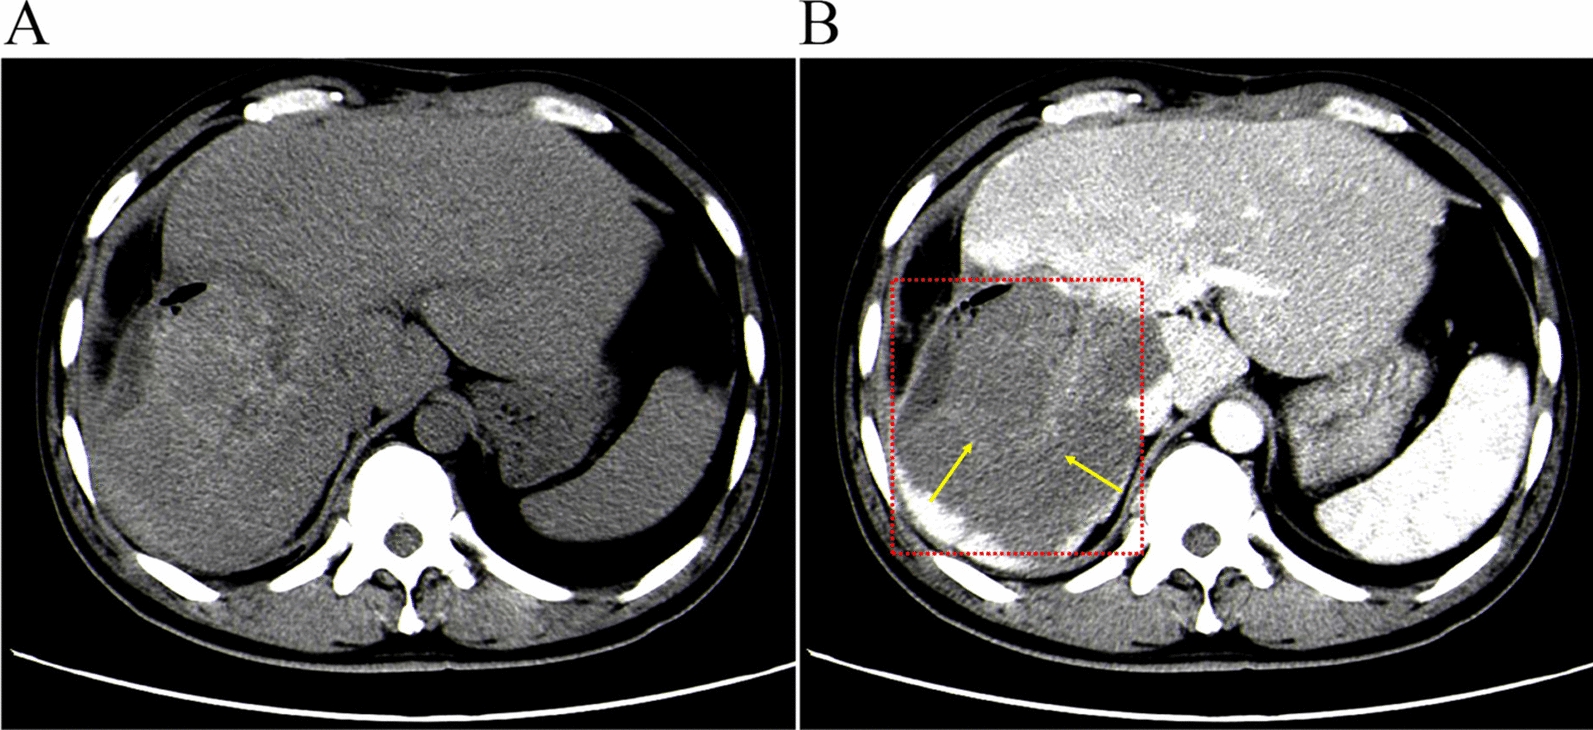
Imaging findings of liver lesions. A Nonenhanced scan image of the liver; B Enhanced scan image of the liver. Abdominal dynamic computed tomography (red arrow) revealed round low-density shadows in the right lobe of the liver, with no enhancement observed following the contrast-enhanced scan (yellow arrow). A mass of mixed density was identified on the right side of the liver, characterized by low soft tissue density, some calcification, and local hepatic indentation. A hybrid mass measuring 11.6 × 11.2 cm^2^ was noted in the right posterior lobe of the liver (red box)
